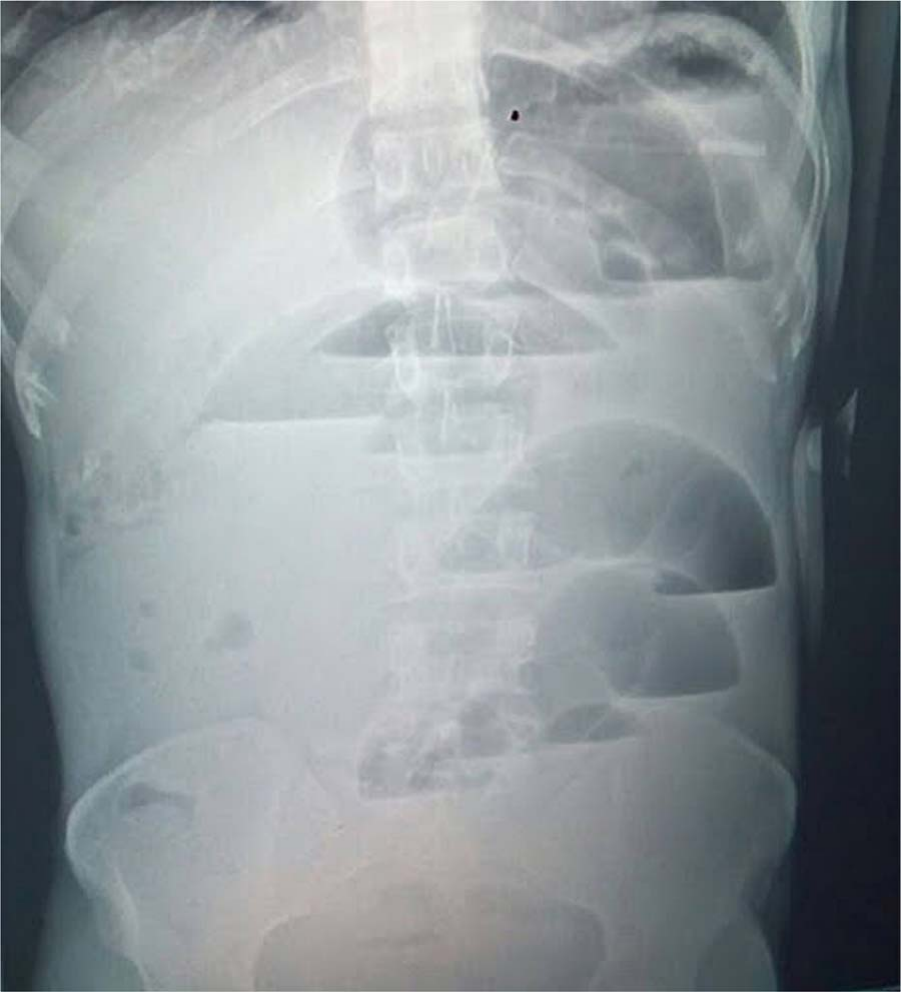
Erect abdominal radiograph showing multiple air–fluid levels and dilated bowel loops.
